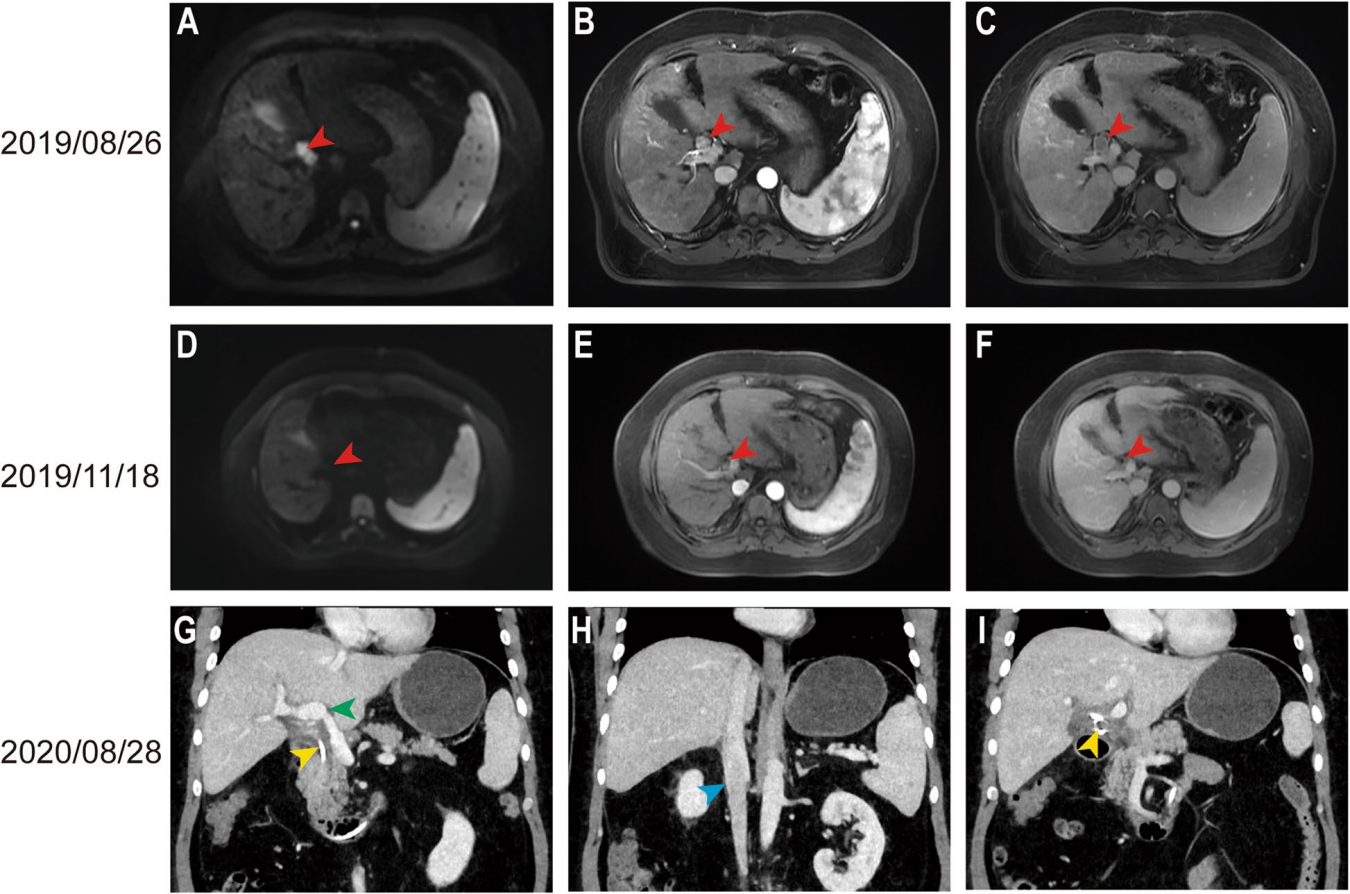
Imaging of patient B before and after treatment. A–C MRI findings at the time of recurrence. The red arrows indicate the lesion. D–F Results of MRI re-examination after 12 weeks of combined medication. The red arrow indicates the original lesion site. G–I CT scan at 6 months after liver transplantation. The green arrow indicates the portal vein, the yellow arrow indicates the bile duct, and the blue arrow indicates the inferior vena cava
